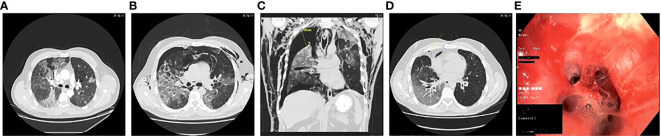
Computed tomography scans showing pulmonary infiltrates, pneumothorax and subcutaneous emphysema at (A) D50; (B) D70; (C) D93; (D) D153; and (E) clinical photo from bronchoalveolar lavage showing mucoid impaction at D63.
